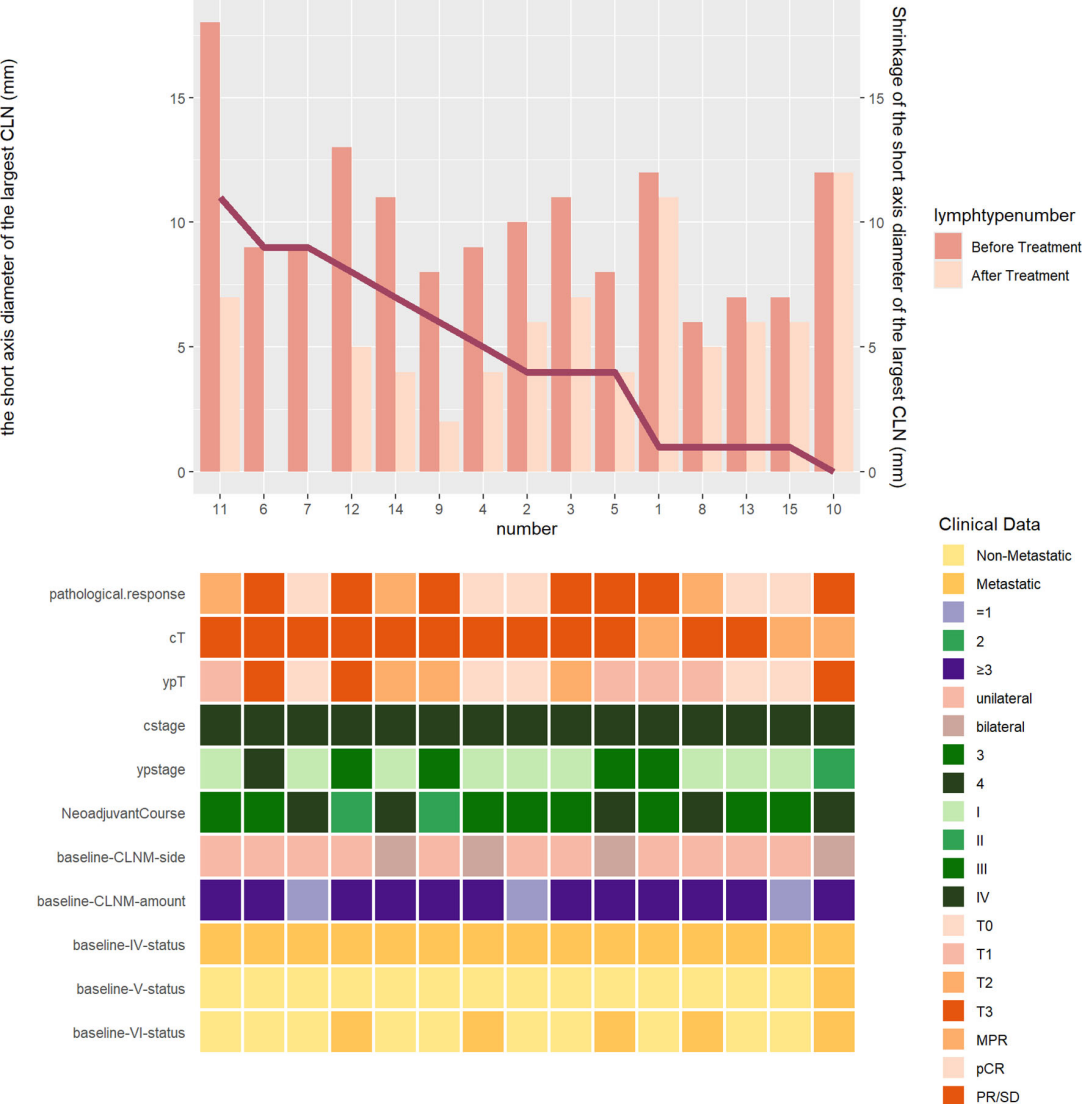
Ultrasound assessment of CLNM short-axis diameter shrinkage before and after neoadjuvant therapy in esophageal cancer. CLNM, cervical lymph node metastasis; cstage, clinical stage; cT, clinical T stage; ypstage, post-neoadjuvant therapy pathological stage; ypT, post-neoadjuvant therapy pathological T stage; ypN, post-neoadjuvant therapy pathological N stage.
